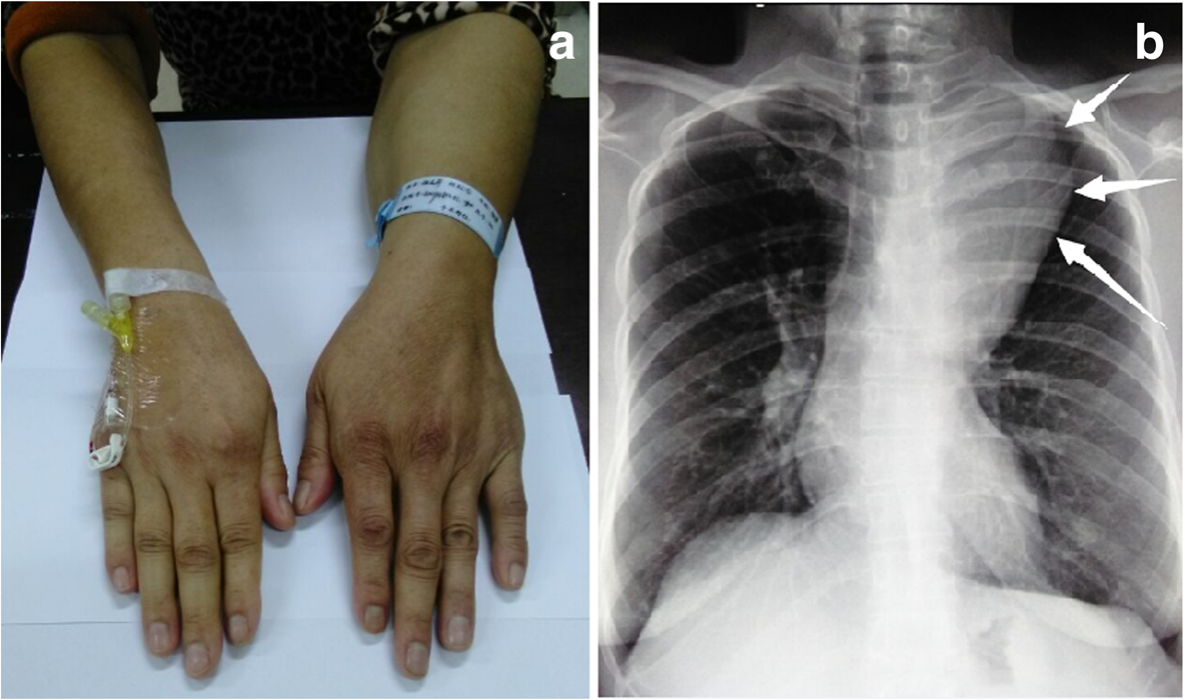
a The left arm muscles were thicker than the contralateral ones. b X-ray of the chest revealed a mass in the superior mediastinum
